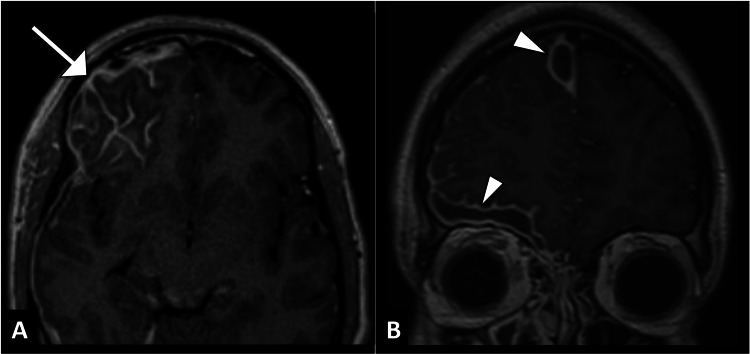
Axial (A) and coronal (B) post-contrast brain MRI images highlight rim-enhancing lesions (arrowhead) and leptomeningeal enhancement (arrow), confirming the presence of subdural empyema in the context of meningoencephalitis. MRI: magnetic resonance imaging
